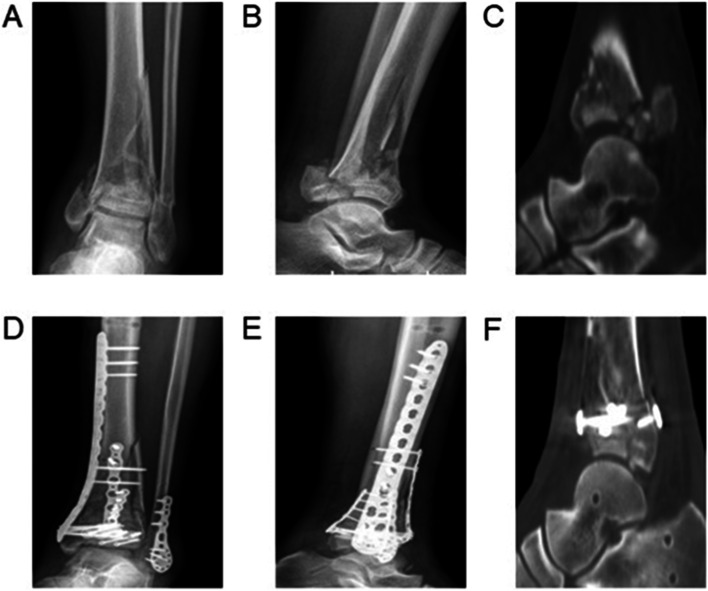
A 58-year-old man involved in a motor vehicle accident developed AO/OTA C3 pilon fracture. A & B) Preoperative anteroposterior and lateral radiographs showed the fibular fracture and distal tibial articular surface comminuted fracture. C) Preoperative sagittal computed tomography (CT) scans showed comminuted fracture of the posterior column articular surface. D & E) The anteroposterior and lateral radiographs showed good reduction and fixation after open reduction and internal fixation through the combined posterolateral and anterior extensile approach. F) Postoperative sagittal CT scans showed that the congruency of the articular surface was not satisfactory
